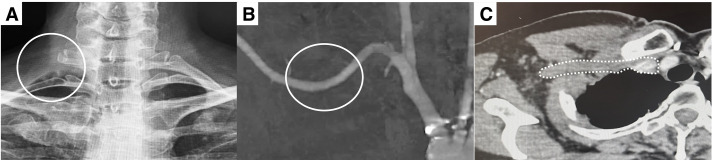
Postoperative images. (A) Radiograph of the first rib. (B) Computed tomography with the arm elevated showing improvement of subclavian artery stenosis at the costoclavicular space. (C) Computed tomography showing improvement of subclavian vein stenosis at the costoclavicular space.
